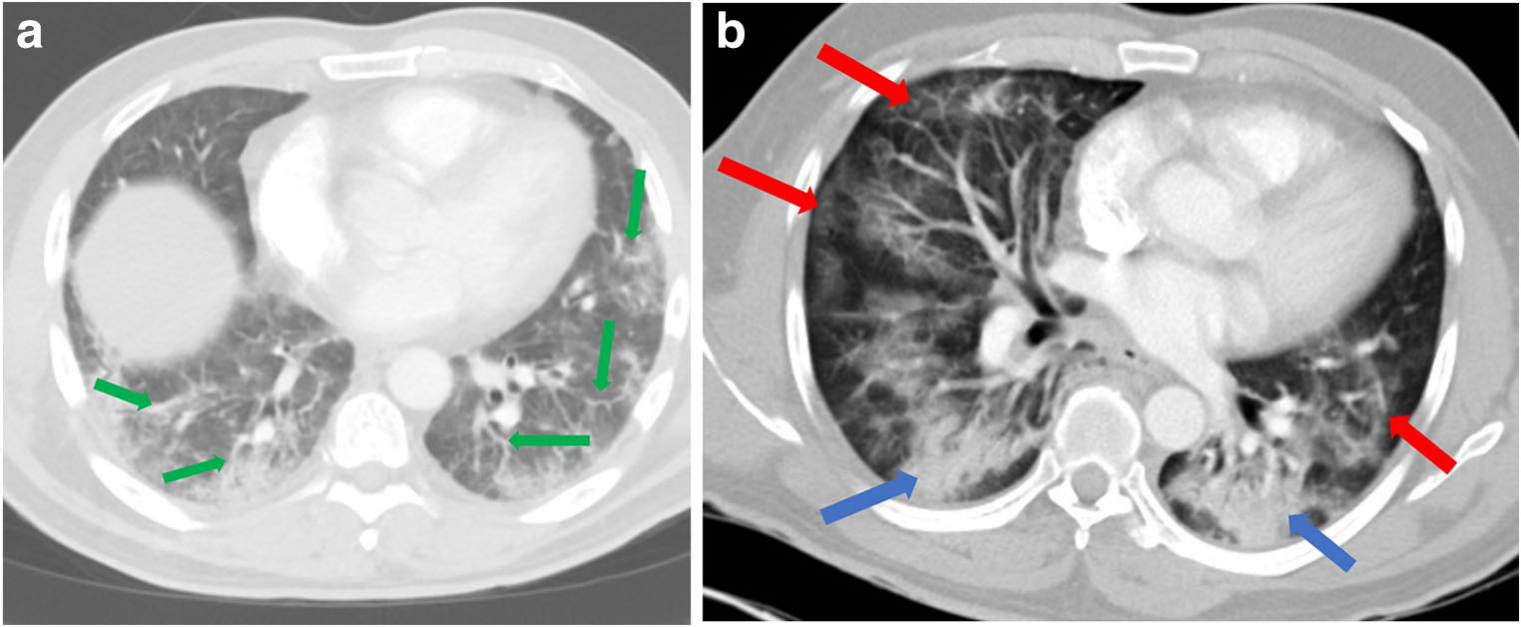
Peak stage COVID-19 (9 to 13 days): a Axial CT scan of the chest in a positive 49-year-old man with COVID-19 demonstrates ground glass opacities (GGO) in a multilobe distribution (red arrows) and bibasilar consolidations (blue arrows). b Diffuse crazy-paving pattern (GGO with superimposed inter- and intralobular septal thickening), consolidations, and residual parenchymal bands (green arrows).
